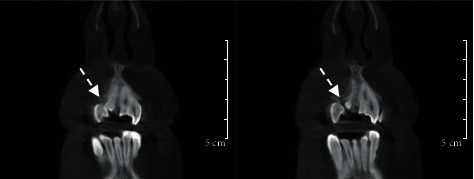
Plane of coronal CBCT sections of maxillary showing the separation level of the fused #11 and #12 (arrow).
